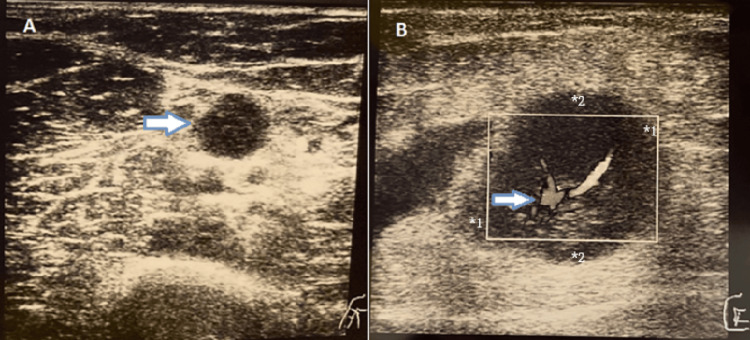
Right elbow ultrasound. Elbow ultrasound showed (A) an adenomegaly (arrow) with diffuse hyperechogenicity of the tissues and (B) an intensely vascularized adenomegaly (arrow) - diameter 1: 22.2 mm, diameter 2: 18.4 mm.
